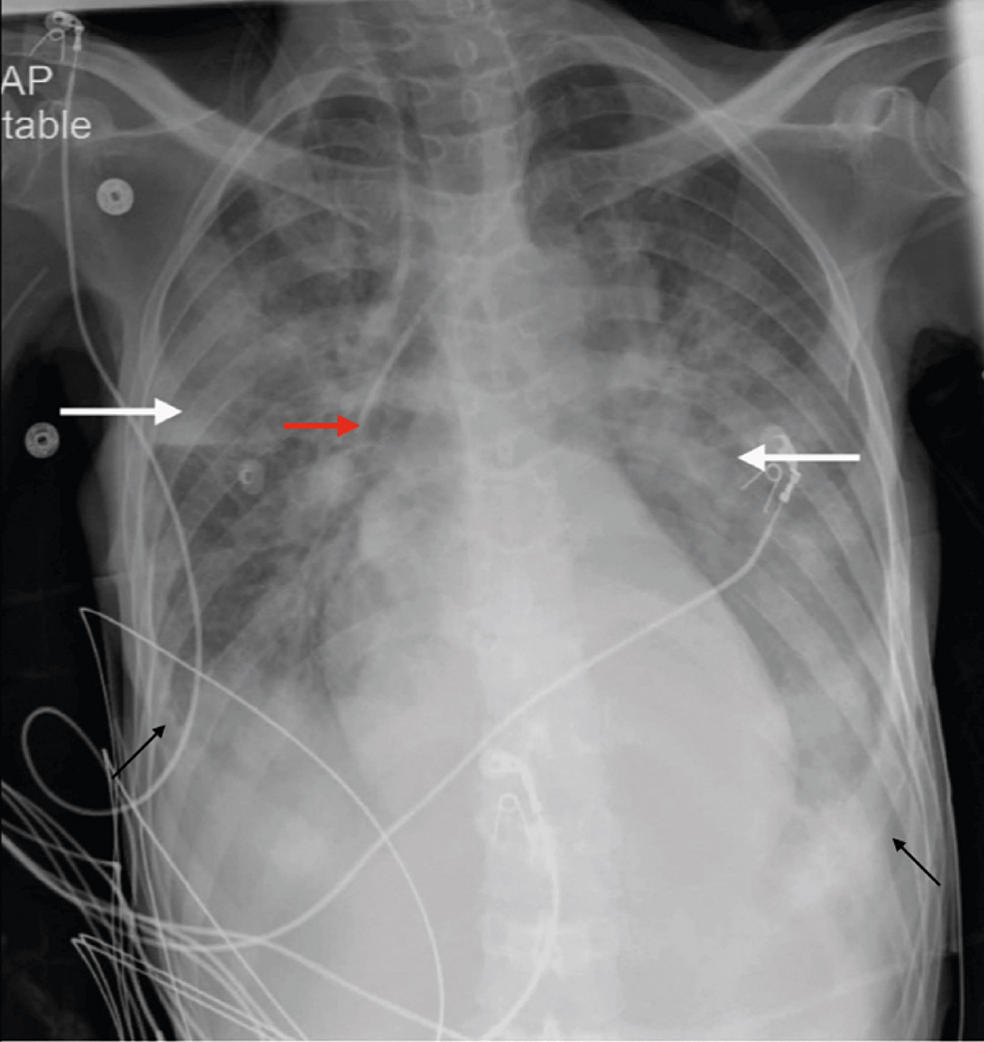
Chest x-ray taken in the ICU showing bilateral pulmonary edema. Chest x-ray was taken in the intensive care unit and read as follows: a left IJ central line catheter is placed, terminating in the projection of mid-SVC (red arrow). Hyperinflated lungs are seen. Widespread patchy alveolar interstitial ground-glass opacities in both lung fields are seen (both white arrows). Likely small bilateral pleural effusion present (black arrows). SVC: superior vena cava.
